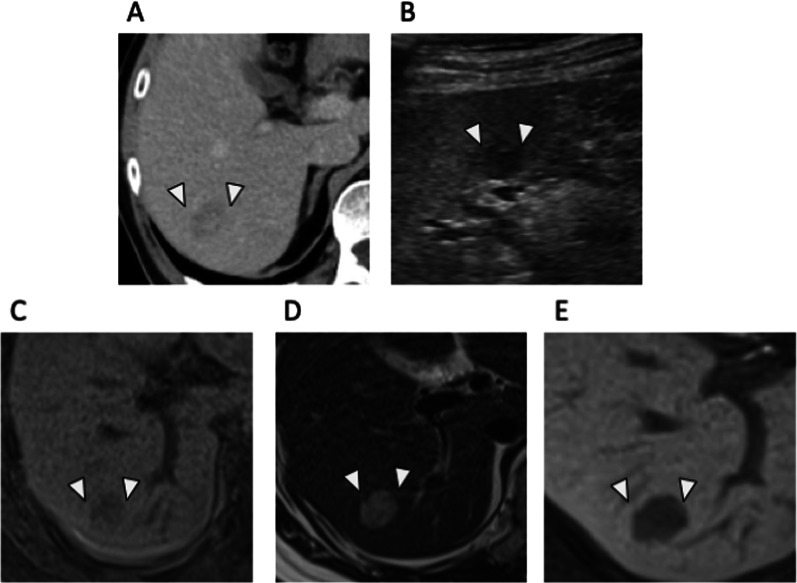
Imaging studies of the liver lesion. A Contrast-enhanced abdominal computed tomography demonstrating a 20-mm, low-density area in hepatic S6/7 in the portal vein phase. B Abdominal ultrasound showing a heterogeneous, internal mass in the hepatic S6/7. C EOB-MRI, low signal intensity on T1-weighted imaging. D EOB-MRI, high signal intensity on T2-weighted imaging. E EOB-MRI, low contrast uptake in EOB-hepatocyte phase
